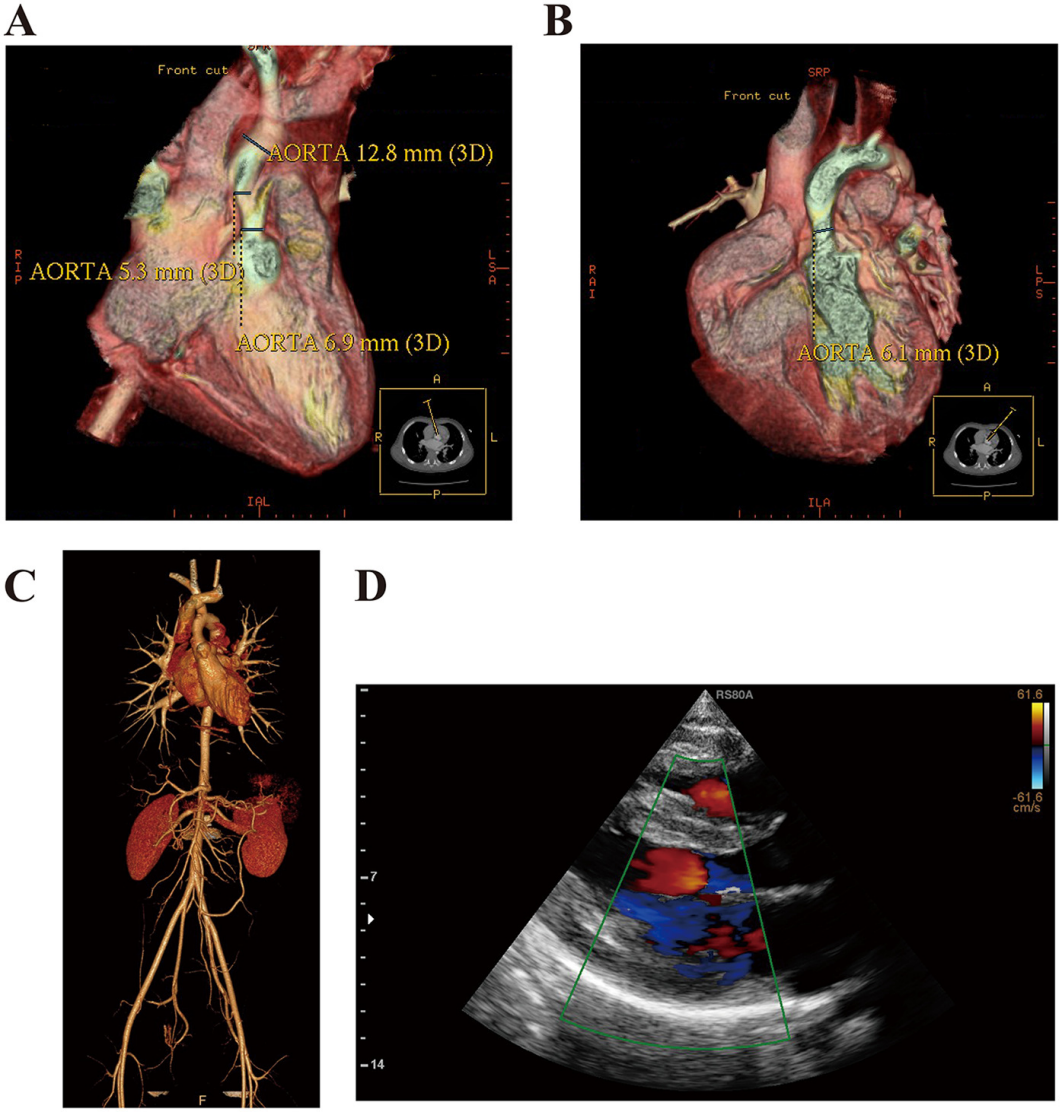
Case presentation and ancillary examination. (A,B) Thoracic aorta CTA confirmed the presence of SVAS in this patient at the age of 2 years. (C) Thoracic and abdominal aorta CTA confirmed that the aortic stenosis was cured after the surgery. (D) Echocardiography revealed the features of HCM. (IVS, interventricular septum; LVPW, left ventricular posterior wall; LVEDD, left ventricular end diastolic diameter; LA, left atrium; EF, ejection fraction).
